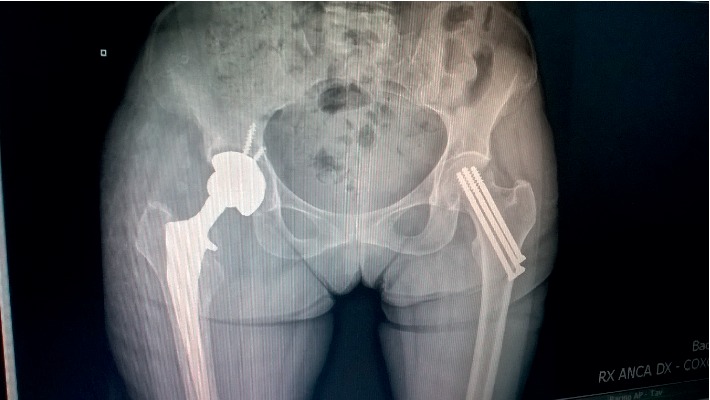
Hip radiography: radiolucency around the acetabular component of the prosthesis.
